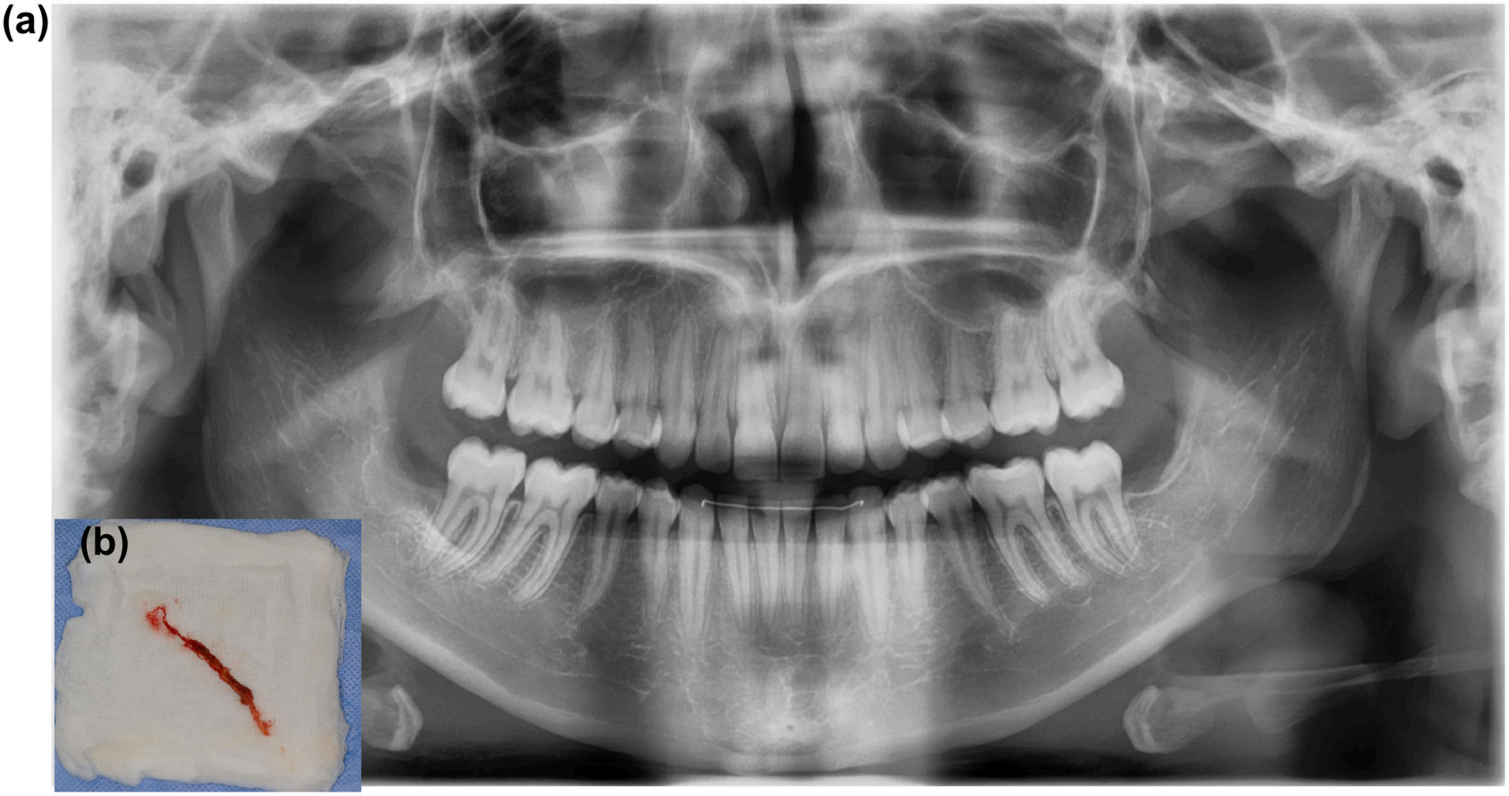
(A) A 16-year-old patient was referred due to persisting pain and pus in the extraction cavity of 48. Radiography shows incomplete bone healing in loco 48. (B) Wound exploration reveals gauze that remained unnoticed in the wound.
